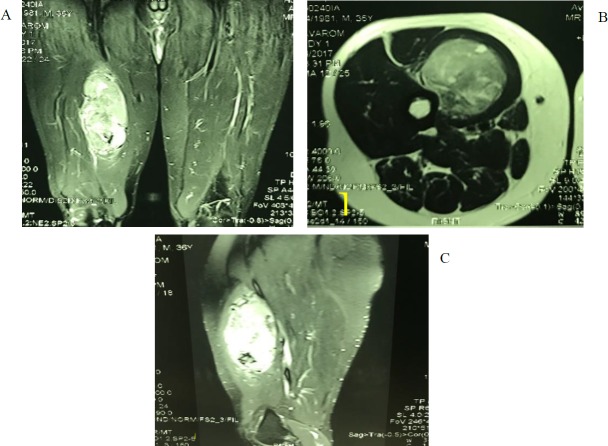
A) AP, B) transverse and C) lateral view of right femur MRI showing large lobulated hyper-vascular mass lesion with the diameter of 16.5X7cm in deep portion of the right rectus femoris muscle
